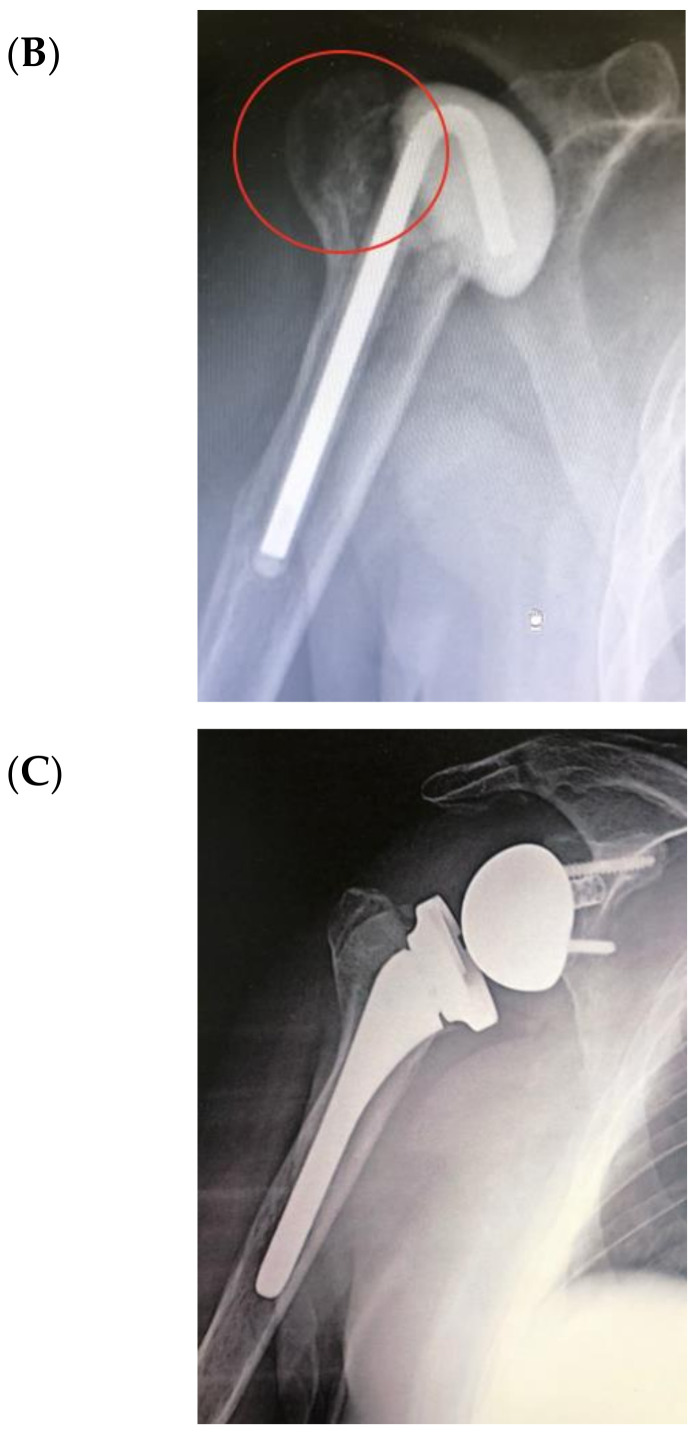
An infection after percutaneous pinning of a proximal humerus fracture. (A) Preoperative X-ray. (B) The subsequent implanting of a cement antibiotic spacer (red circle underlines bone loss); (C) the final reverse arthroplasty (RSA) with the addition of bioactive glass in the tuberosity defect.
